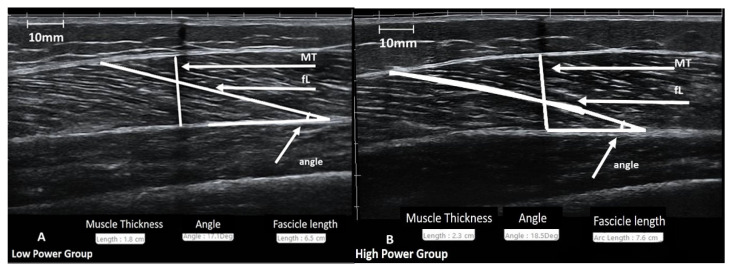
Representative images from the ultrasographic evaluation of vastus lateralis muscle for the Low-Power (A) and High-Power groups (B).
